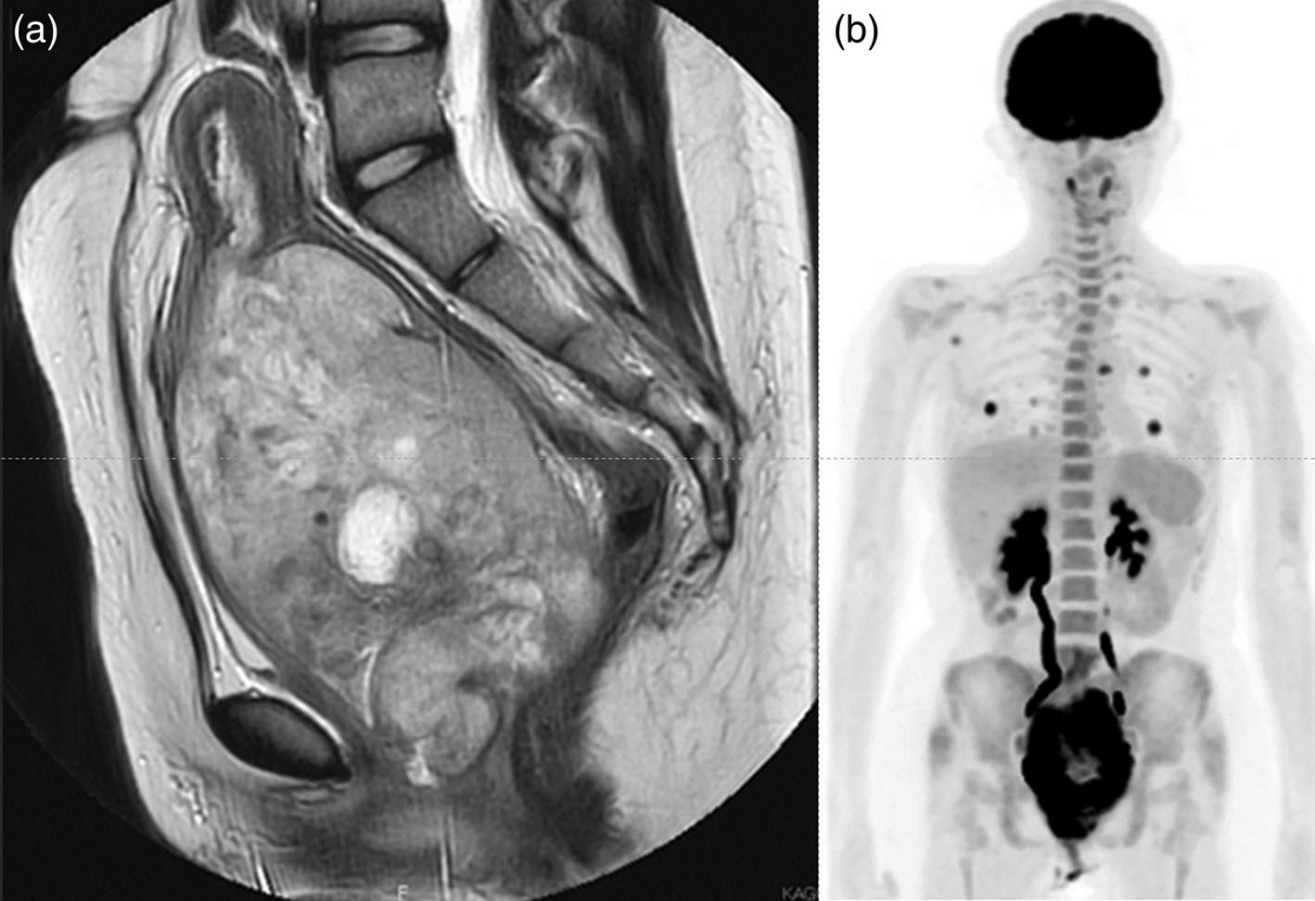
Magnetic resonance imaging with T2‐weighted images show (a) a large 14 × 10 cm tumor with high intensity in the cervix. (b) Positron emission tomography‐computed tomography revealed fluorodeoxyglucose accumulation in the cervical mass, multiple lung nodules, and the right scapula.
